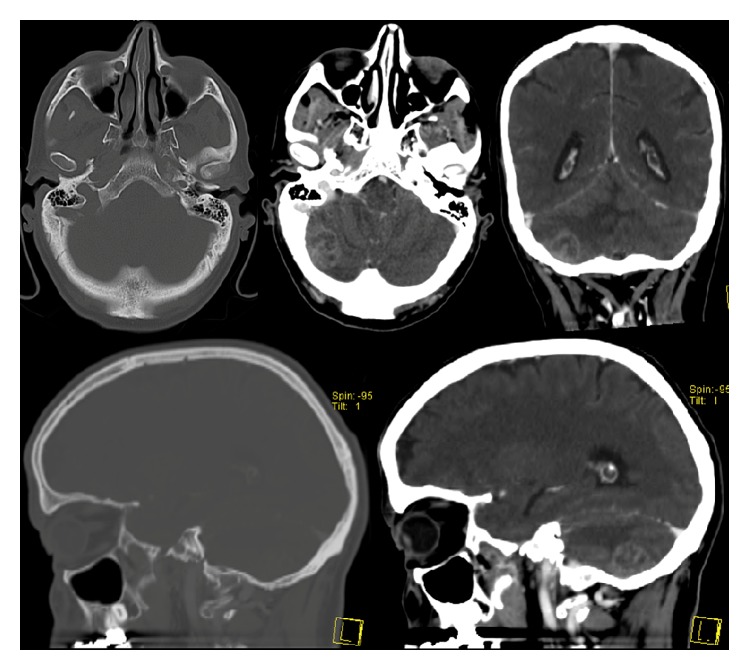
Brain computed tomography scan (bone and brain windows) showing one lesion in the right cerebellum with heterogeneous contrast enhancement.
